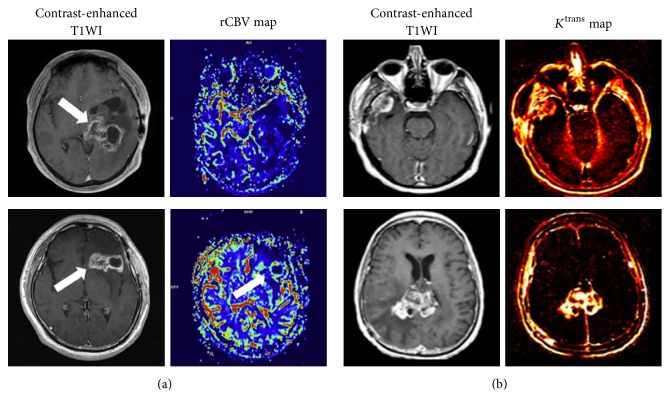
Discrimination of PsP from PD using DSC-MRI and DCE-MRI. (a) Contrast-enhanced T1WI of GBM treated with temozolomide demonstrates increased contrast enhancement suspicious for both PsP* (top row)* and PD* (bottom row)*. Corresponding rCBV maps show low perfusion in PsP and high perfusion in PD; (b) *K*^trans^ maps demonstrate decreased *K*^trans^ value in PsP* (top row)* compared with PD* (bottom row)*. Reproduce with permission from Shin et al. [158] and Thomas et al. [161].
